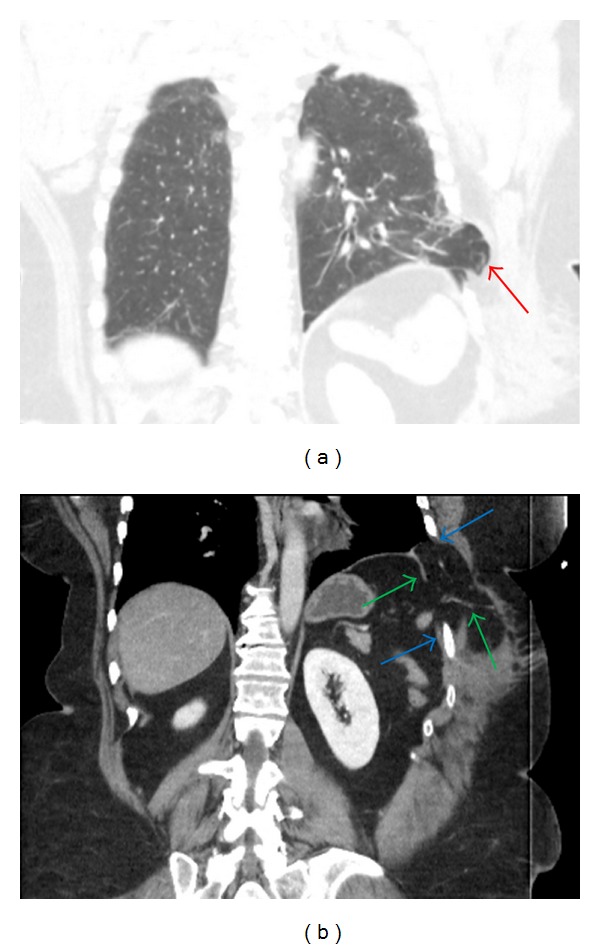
Coronal CT images of the abdomen demonstrate herniation of lung beyond the thoracic cavity (red arrow) (a). There is discontinuity of the diaphragm (green arrows) and widened intercostal space (blue arrows) (b).
